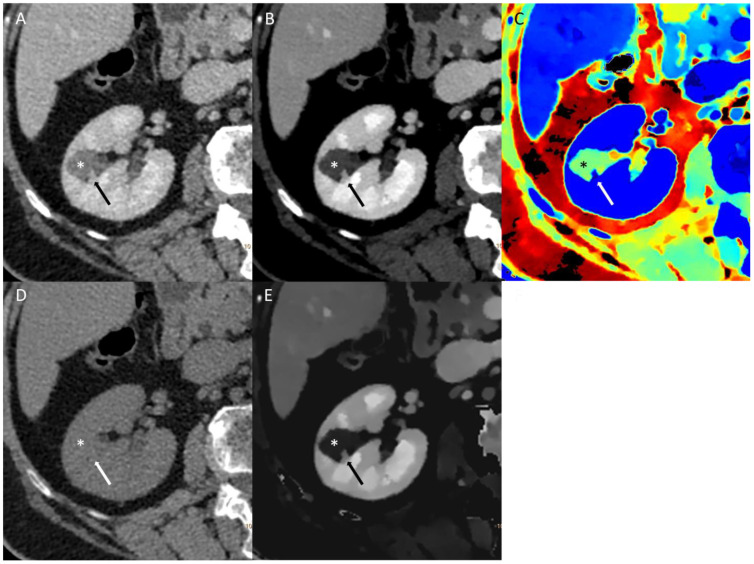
Conventional multi-energetic CT image in portal venous phase (A) of a small dense renal cyst (*) with a millimetric polypoid component (arrow). The cyst has a 49.2 HU density, thus barely visible in this image, so it is impossible to distinguish between a dense cyst and a hypovascular renal lesion. The 40 KeV Virtual Monoenergetic Image (B) shows poor changes in the enhancement of the cystic component and a good increase in the density of the polypoid component. Z-effective maps image (C) shows low atomic numbers within the cystic component and high numbers in the polypoid component. Virtual Non-Contrast image (D) shows no difference in the densitometry of the cystic component. Iodine density imaging (E) shows no iodine concentration in the cystic component and iodine concentration in the polypoid component. The lesion was classified as a class III Bosniak renal cyst, and afterwards, a multidisciplinary meeting was decided on for follow-up (mainly for dimensions). A control CT scan at 12 months (not shown) demonstrated no differences.
